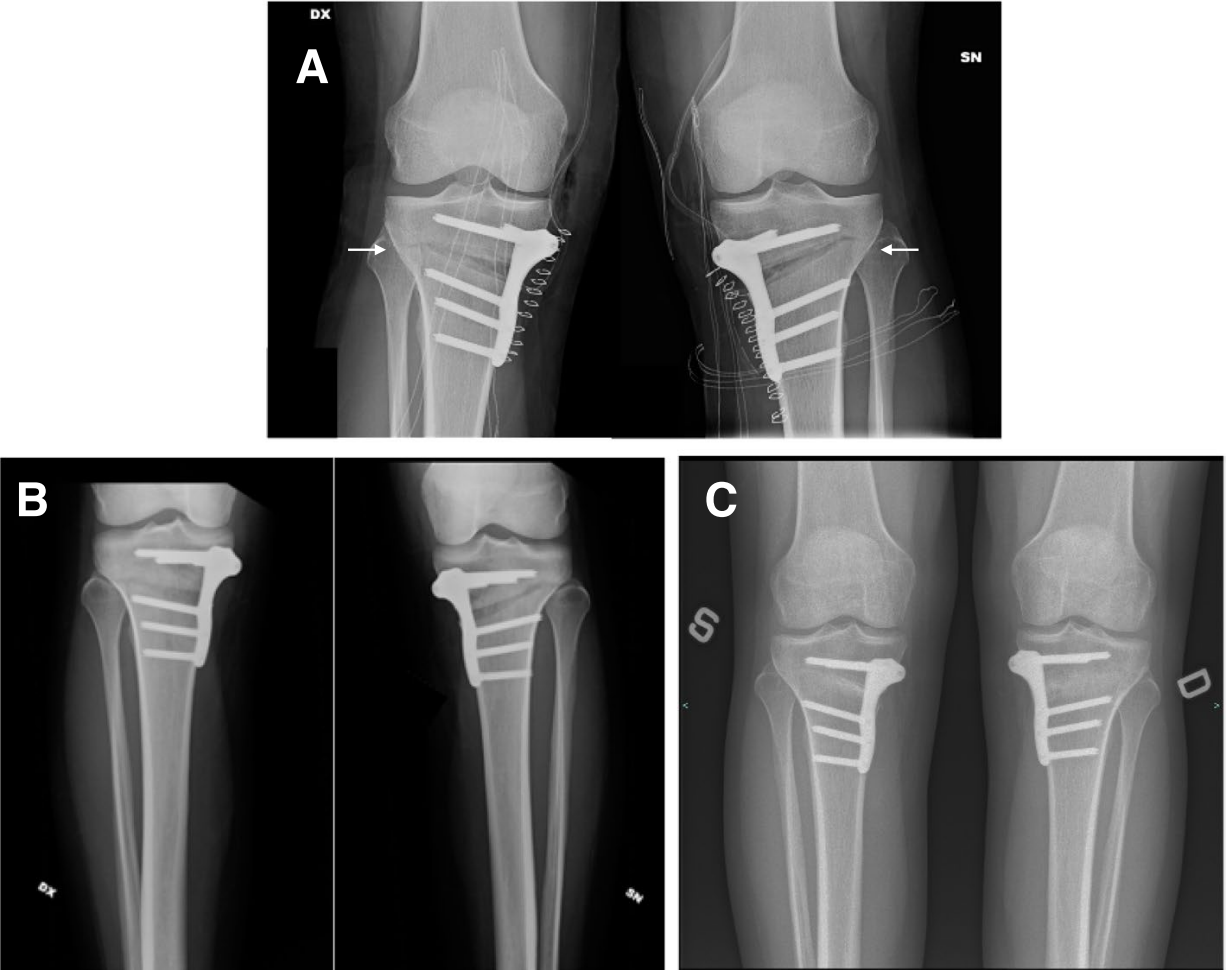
Radiological progression of gap healing. A Postoperative x-Ray of SBHTO showing a type 2 Takeuchi hinge fracture. (white arrows). B X-rays at 2 months postoperative. No displacement. Bone callus is evident up to zone 4 bilaterally. C X-ray at around 6 months after surgery shows complete bone healing bilaterally
